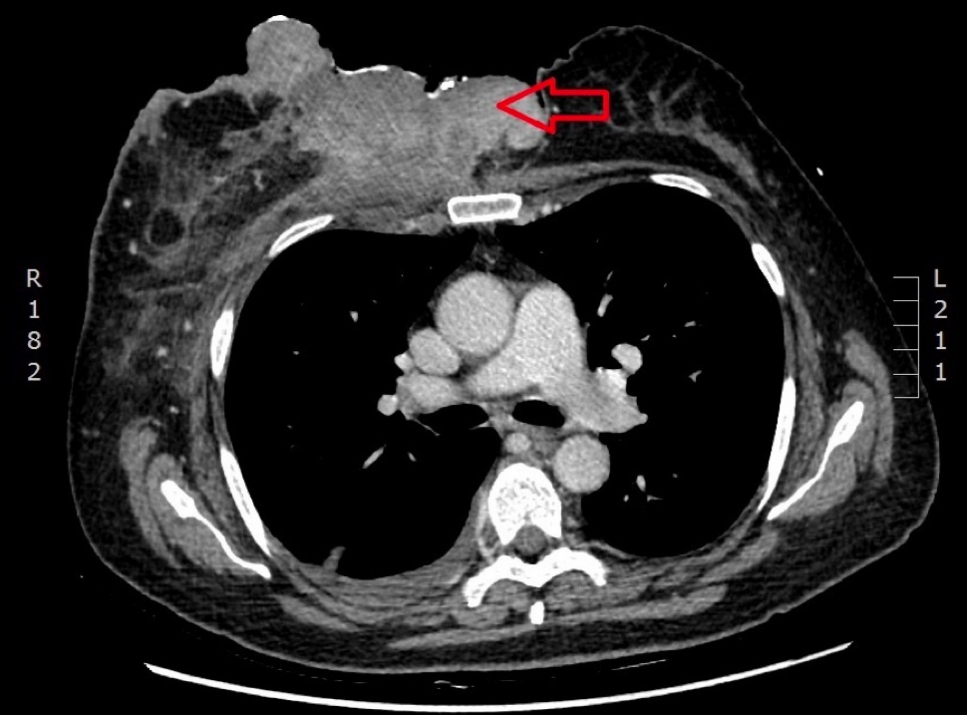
CT scan before treatment Large tumor mass fixed to the chest wall (*arrow*)
